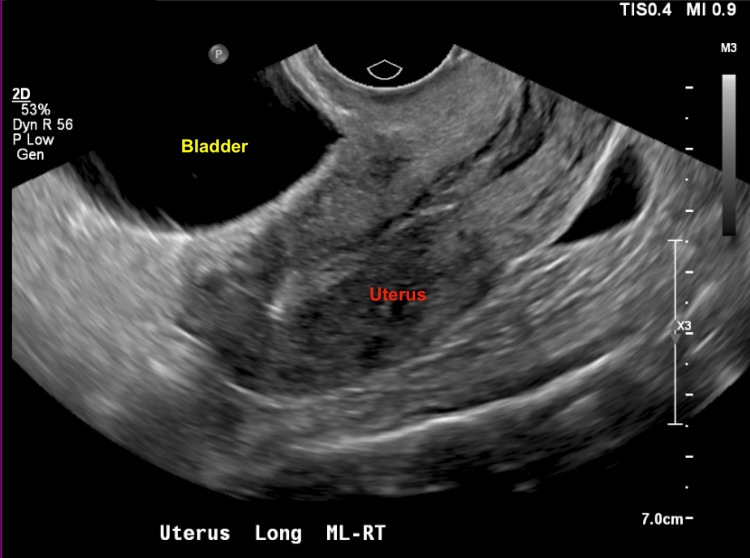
Transvaginal ultrasound demonstrating the uterus and bladder No visible mesothelial cyst is noted in this view, contrasting with the presence of the mesothelial cyst seen in the corresponding CT scan.
